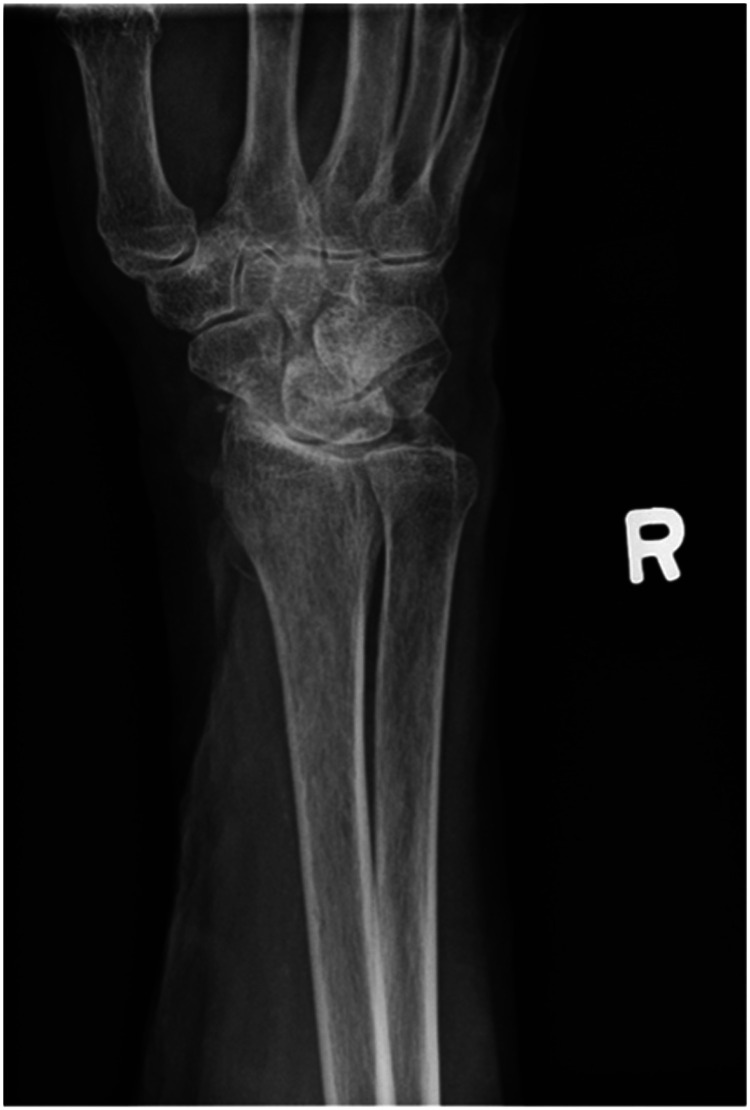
Preoperative right hand plain film oblique view x-ray.
